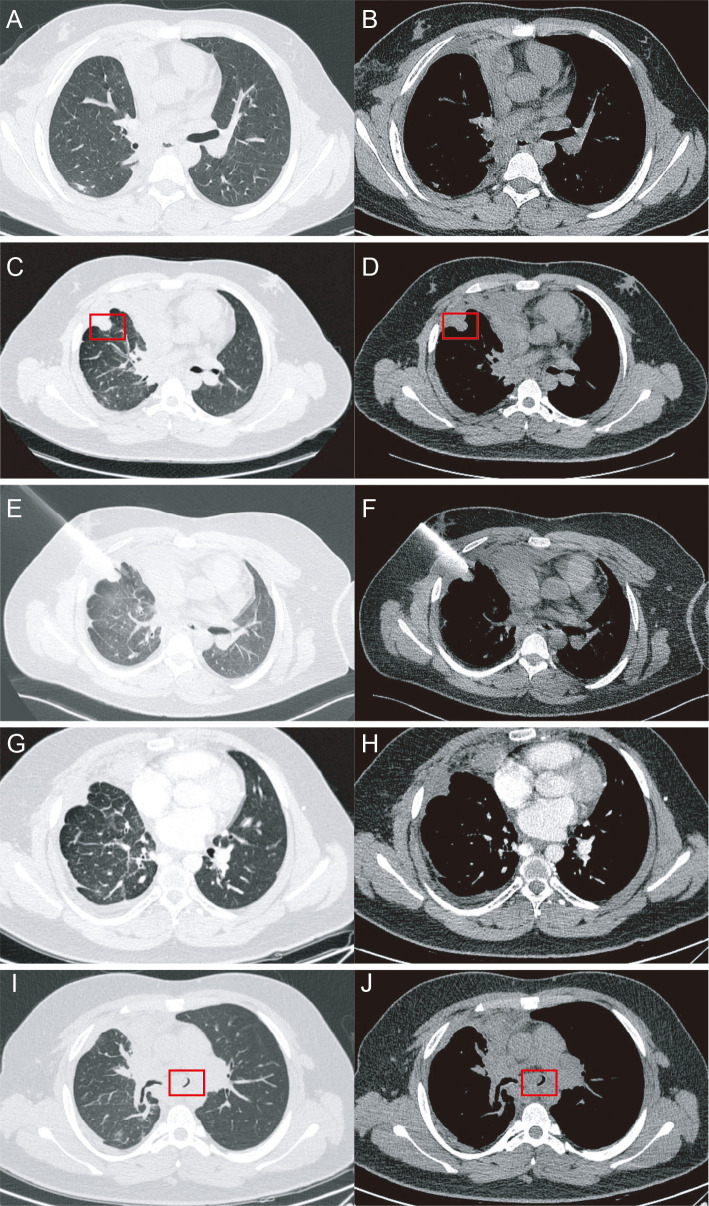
Chest CT images. 1-month re-examination after surgery (A), Lung window. (B), Mediastinal window). 22-month re-examination after surgery (C), Lung window. (D), Mediastinal window). During ablation (E), Lung window. (F), Mediastinal window). 3-month re-examination after ablation and radiotherapy (G), Lung window. (H), Mediastinal window). 3-month re-examination after nab-paclitaxel plus camrelizumab therapy [(I), Lung window.(J), Mediastinal window].
